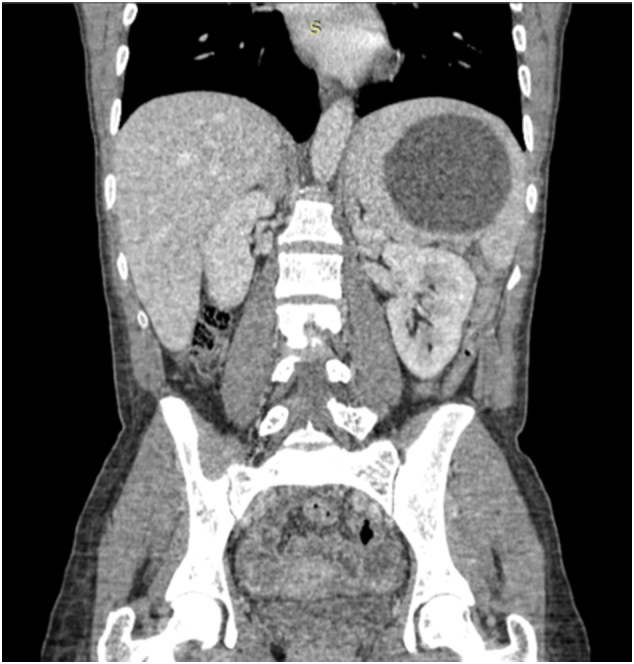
CT coronal view of a primary splenic hydatid cyst.
